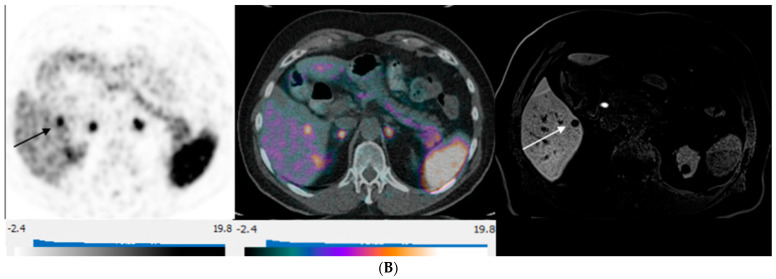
80-year-old man with well-differentiated G2 small bowel neuroendocrine tumor referred for restaging. ^68^Ga-DOTATATE PET/CT and Hepatobiliary-specific contrast-enhanced MR show a similar number and distribution of metastases. (A) Axial ^68^Ga-DOTATATE PET (left), fused PET/CT (middle), and corresponding hepatobiliary phase T1 VIBE image (right) show intense focal radiotracer uptake (arrows) in two small segment 8 metastases. (B) Axial ^68^Ga-DOTATATE PET (left), fused PET/CT (middle), and corresponding hepatobiliary phase T1 VIBE image (right) show intense focal radiotracer uptake in a small segment 5 deposit (arrows).
